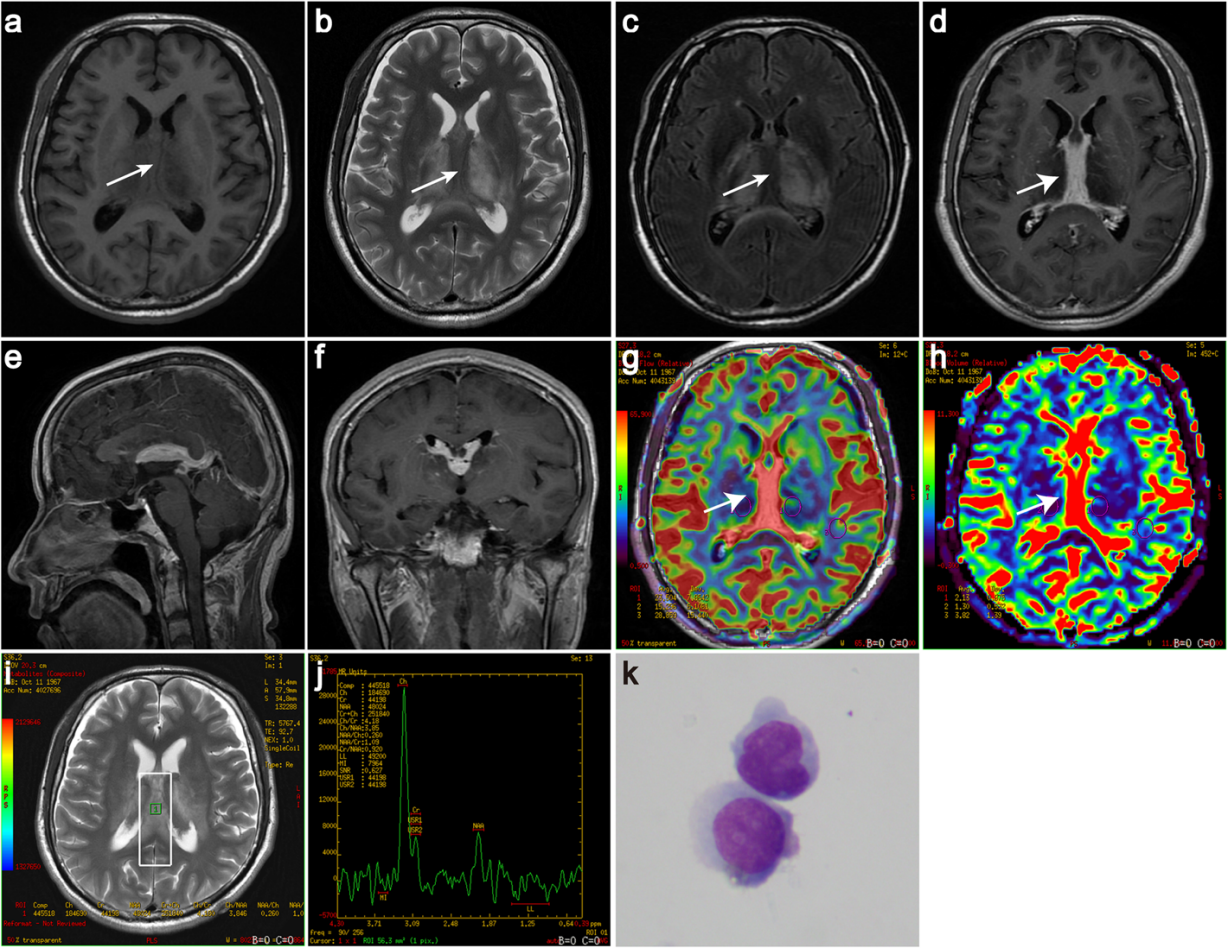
The solid lesions in the bilateral ventricles presenting as isointense on T1WI (a, white arrow) and hypointense on T2WI and FLAIR images (b and c, white arrow). Striking contrast enhancement (d-f, axial (white arrow), sagittal, and coronal T1 with contrast) and hyperperfusion are demonstrated in the lesions of the bilateral ventricles (g and h, white arrow, rCBF, rCBV map). MRS from the lesion demonstrates a high Cho peak and a low NAA peak with a Cho/NAA ratio of 3.85 (i and j). CSF cytology reveals malignant cells (k)
